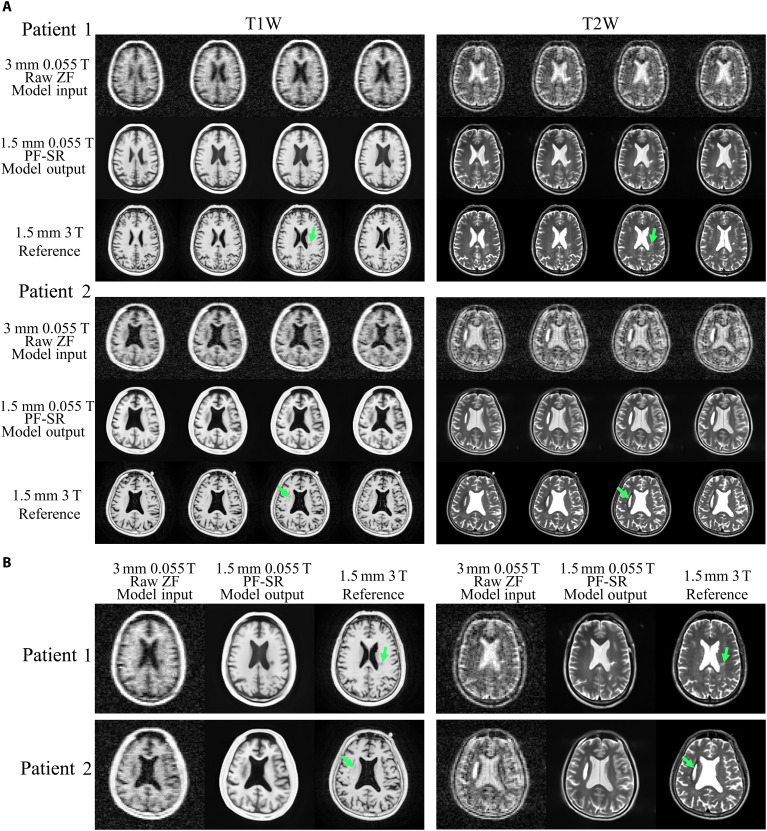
Reconstruction of experimental low-resolution 3D brain data with prospective 2D PF sampling from two patients, acquired from a low-cost shielding-free 0.055-T MRI head scanner, using PF-SR. (A) Patient 1 was a 64-year-old male with chronic (~10 years) ischemic stroke. Patient 2 was a 53-year-old male with right basal ganglia hematoma due to (>2 months) hemorrhage. Four axial slices from PF-sampled low-resolution noisy raw images and PF-SR results are shown for each patient, as well as the corresponding 3 T reference images. (B) Enlarged views of single representative slices. Locations and boundaries of lesion reconstructed by PF-SR were supported by 3 T reference images.
